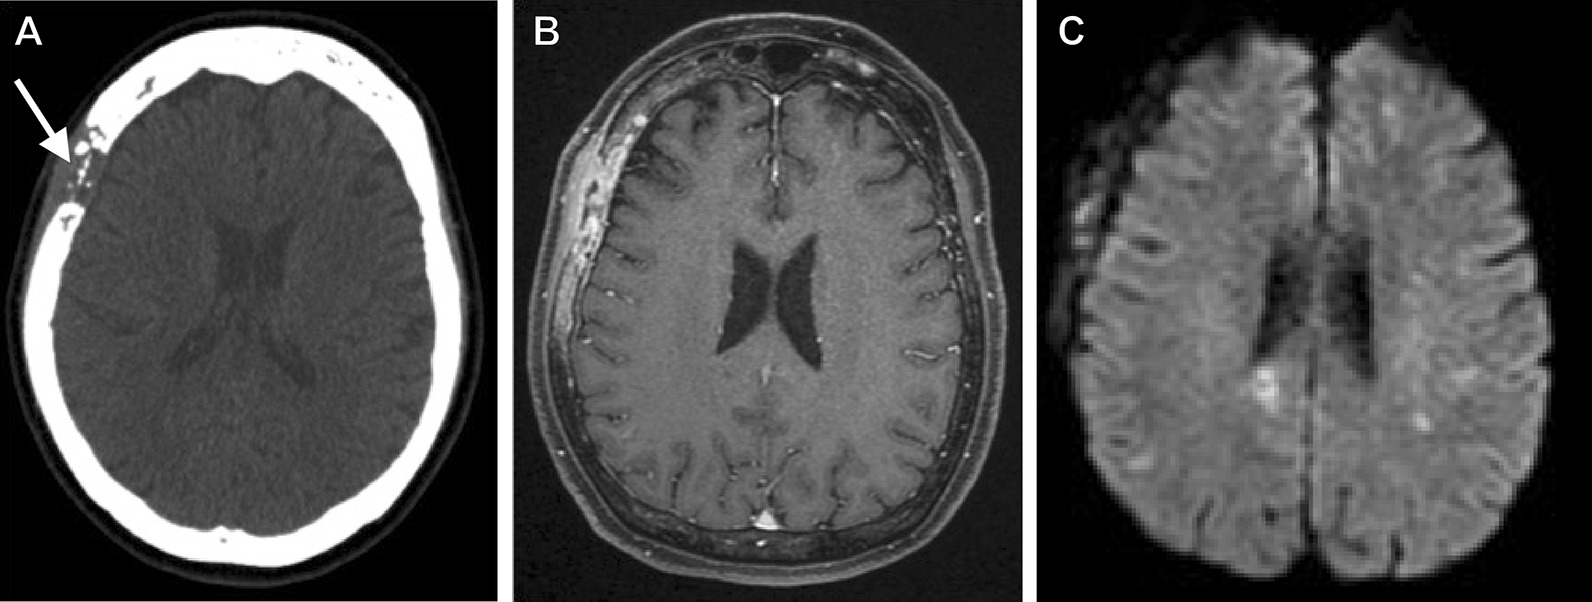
Preoperative images. A Head computed tomography (CT) showing bone destructive skull tumor spreading from the right frontal bone to the parietal and temporal bones (arrow). B Preoperative T1-weighted contrast-enhanced magnetic resonance imaging showing that the legion had invaded the surrounding subcutaneous tissue and temporal muscle. C Diffusion-weighted image showing new multiple cerebral infarctions in the bilateral hemispheres
